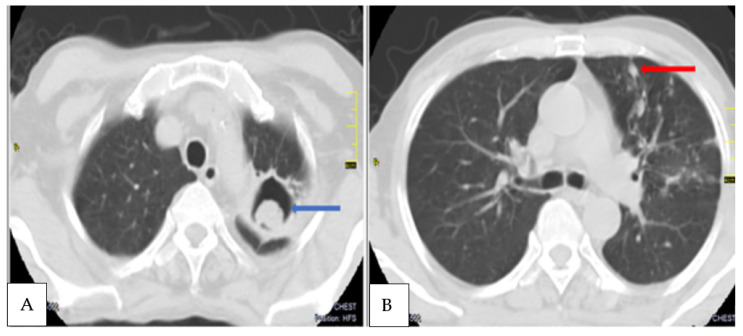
Axial contrast CT scans, axial lung window of chest of another CPA patient diagnosed at T_2_ showing (A) a soft tissue mass within a left apical lung cavity (blue arrow) with a characteristic crescent of air around it, the ‘Monod sign’, indicative of an aspergilloma. (B). Nodular opacities (red arrow) and non pericavitary fibrotic changes in the left upper lobe.
